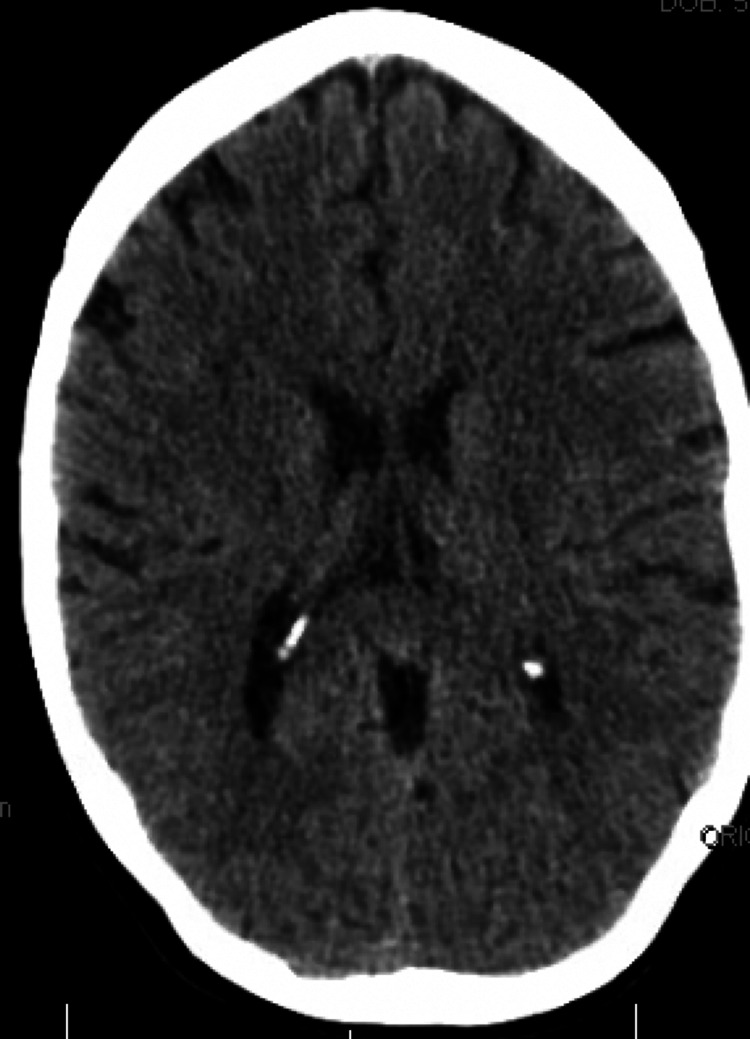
Computed tomography (CT) of the head
